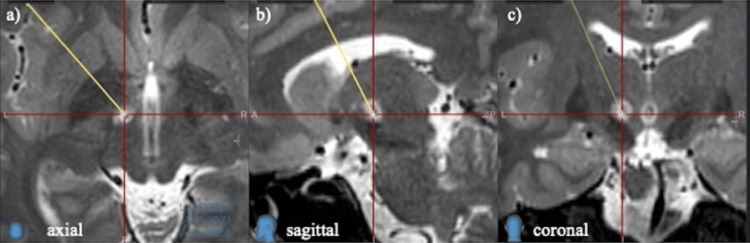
Postoperative MRI Postoperative MRI T2-weighted images in (a) axial, (b) sagittal, and (c) coronal planes are shown. The yellow line represents the planned trajectory, and the intersection of the red lines indicates the planned target.
